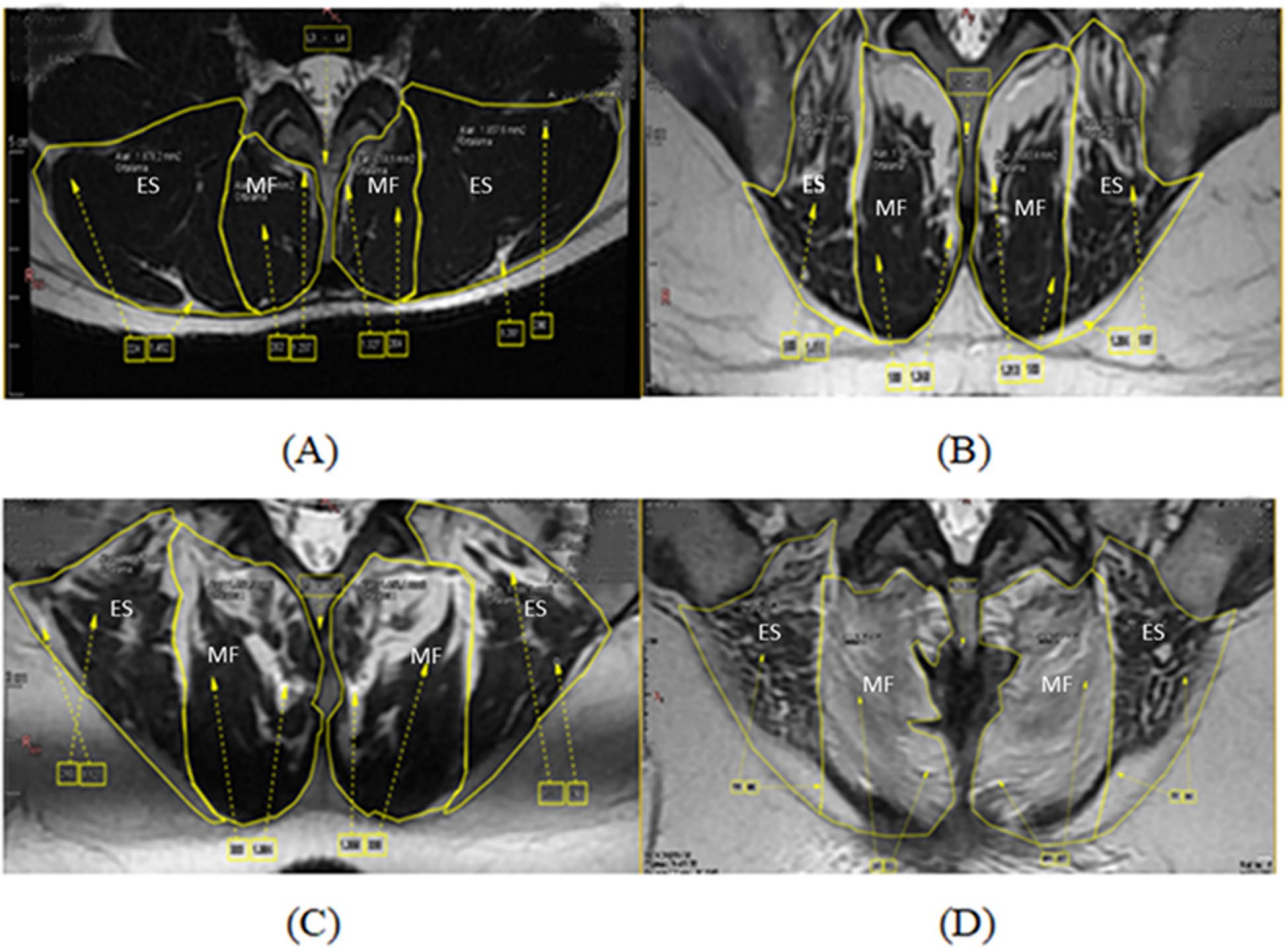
T2 axial magnetic resonance imaging slices showing location of the muscles and degree of fat infiltration, evaluated with the 4-grade system. A Grade 1: < 10% fat infiltration, B Grade 2: 10%- 30% fat infiltration, C Grade 3: 30%-50% fat infiltration, D Grade 4: > 50% fat infiltration, Musculus multifidus (MF), Musculus erector spinae (ES)
